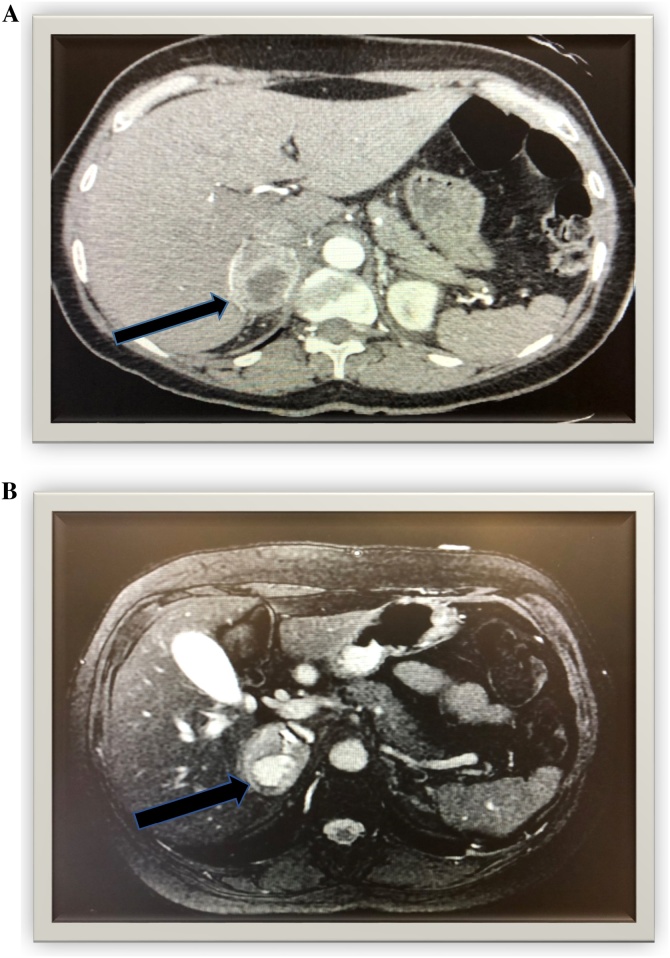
Image A: axial CT imaging with IV and PO contrast showing heterogeneously-enhancing 4 cm right adrenal mass (red arrow) with 2 cm central cystic component. Image B: axial MR imaging with IV and PO contrast of right adrenal mass (red arrow) demonstrating heterogeneous features and avid enhancement of cystic components.
